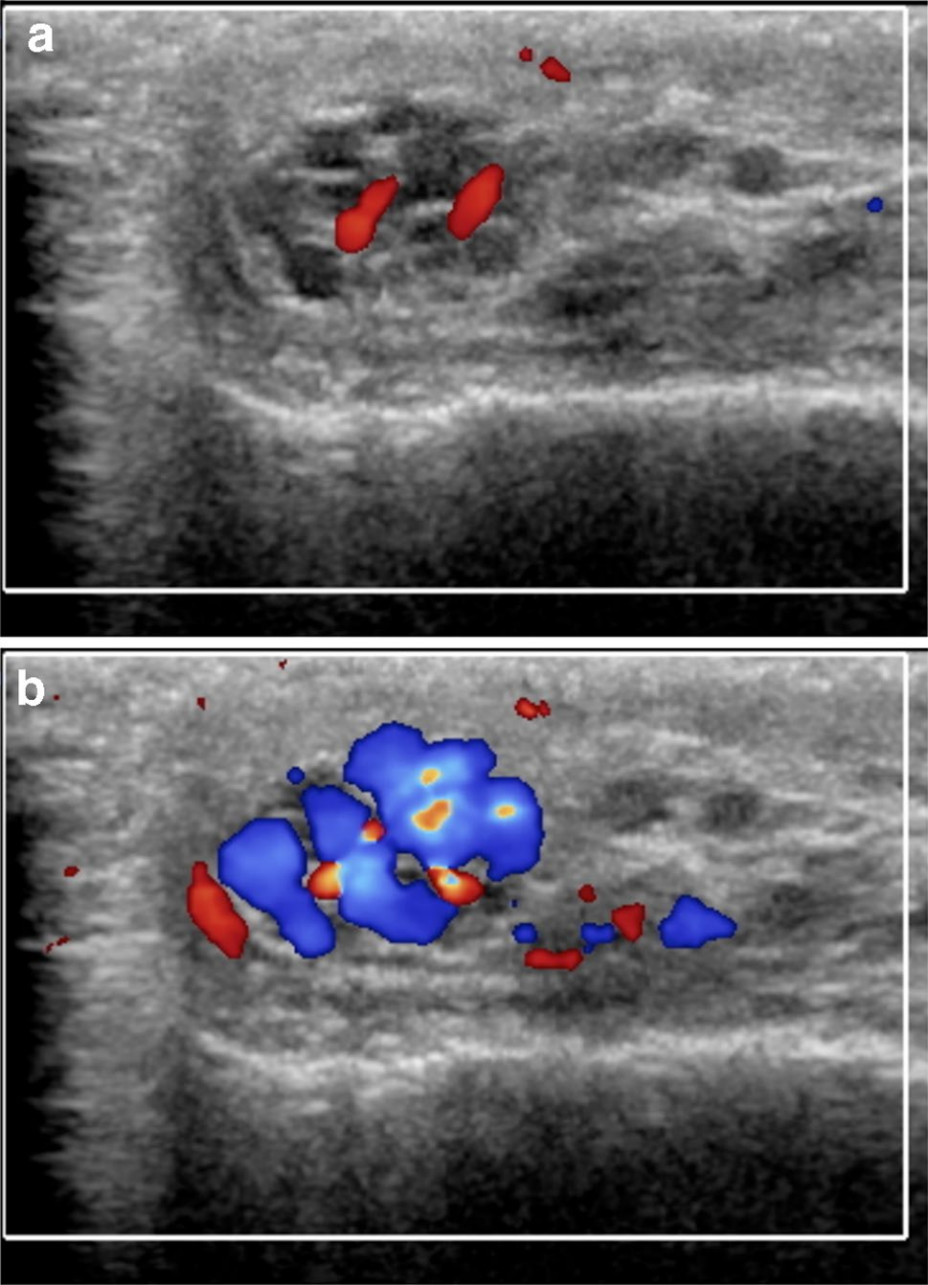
Sarteschi’s grade I varicocele. Colour Doppler images obtained at rest (a) and during Valsalva (b) showing dilated veins of the spermatic cord with reflux during Valsalva at the inguinal canal
